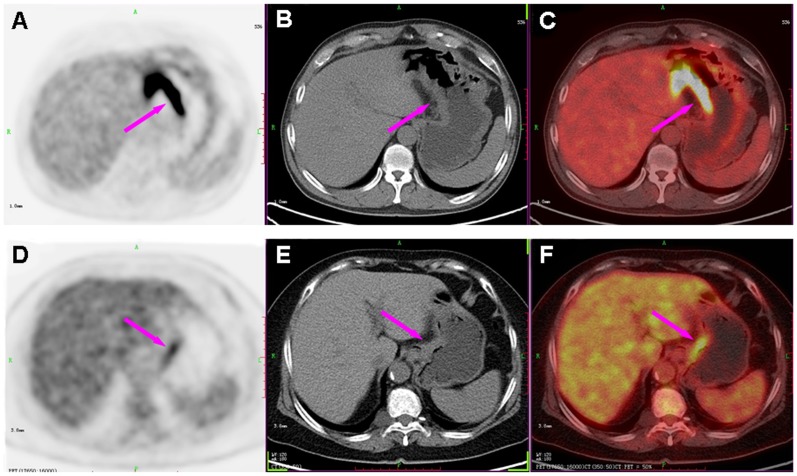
Comparison of PGL and AGC with representative cases. PET (left column), CT (middle column) and PET/CT fused images (right column) of a 56-year-old male with diffuse large B-cell lymphoma (A, B and C) and a 69-year-old female with poorly differentiated AGC (D, E and F), showing gastric lesions in the lesser curvature of stomach with similar thickness of the gastric wall (THKmax: 1.4 vs. 1.6 cm; arrows in B, E). However, the SUVmax was higher for PGL (SUVmax of 22.78; arrow in A) than AGC (SUVmax of 5.24; arrow in D).
